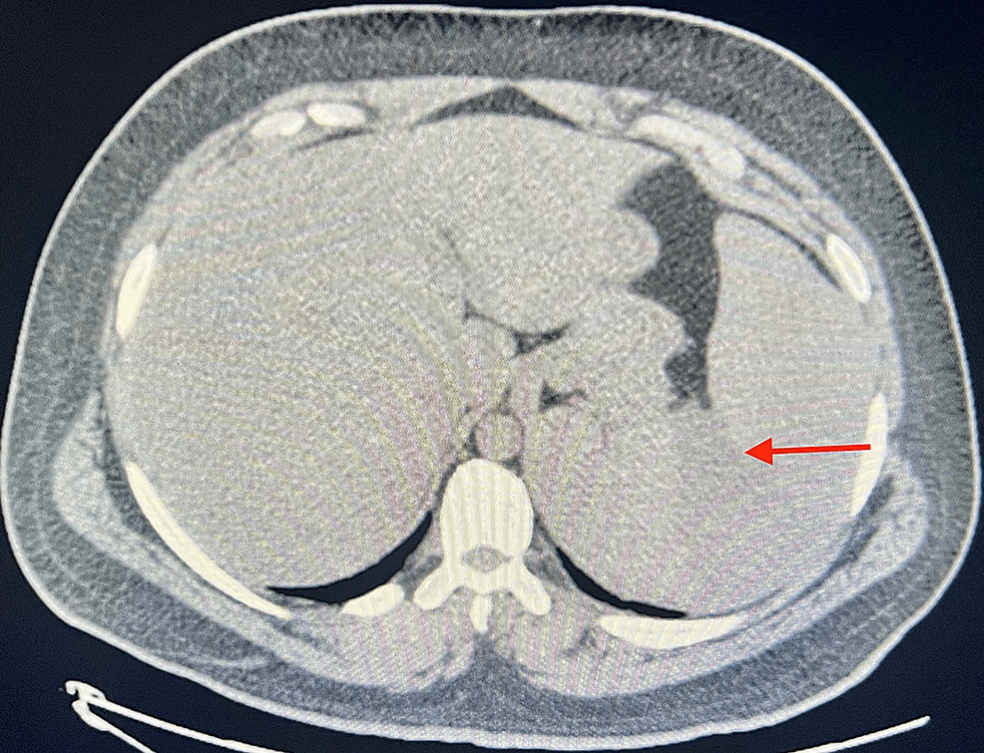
CT of the abdomen and pelvis showing splenic infarct
